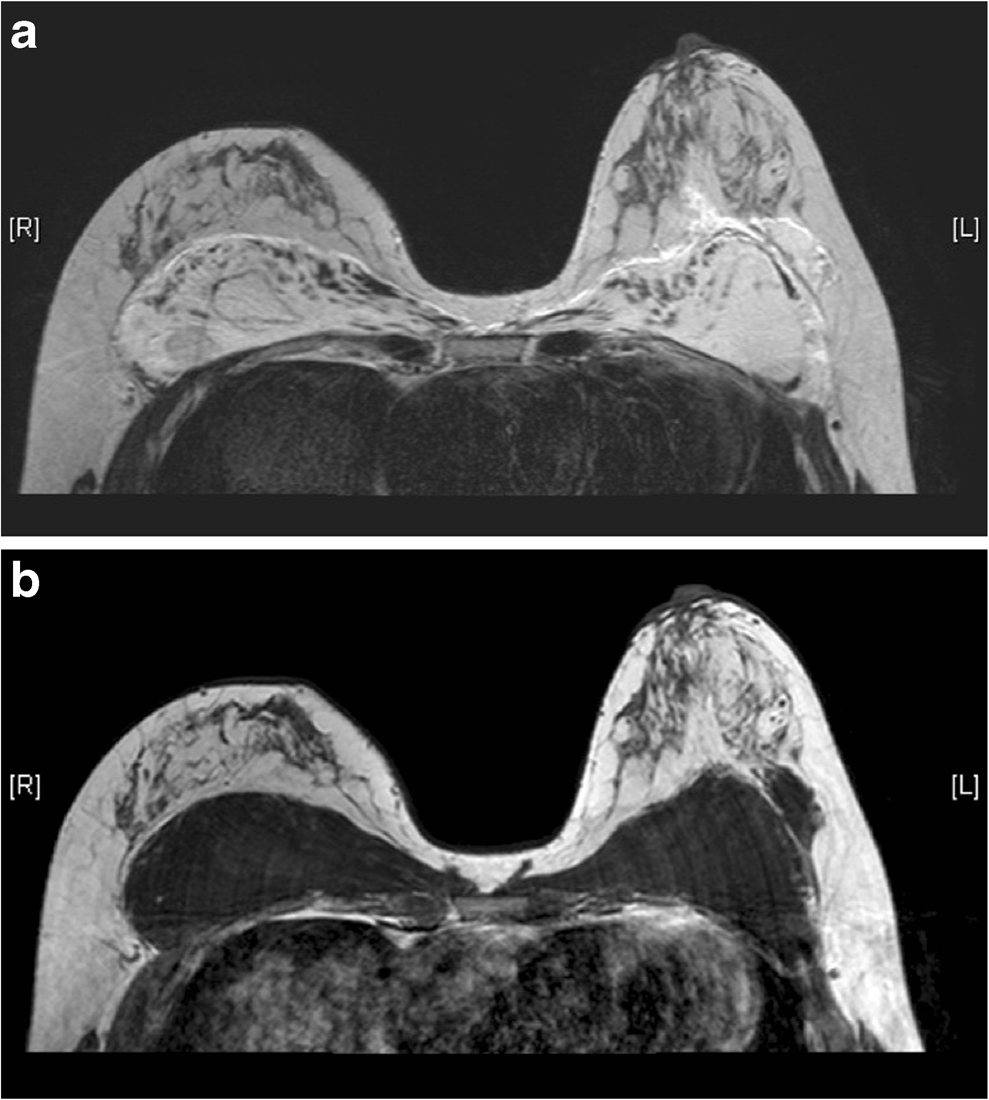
Asymmetric breasts after PAAG injection. T2-weighted (a) and T1-weighted (b) axial MR images show the undesirable outcome of breast asymmetry after augmentation by PAAG injection
